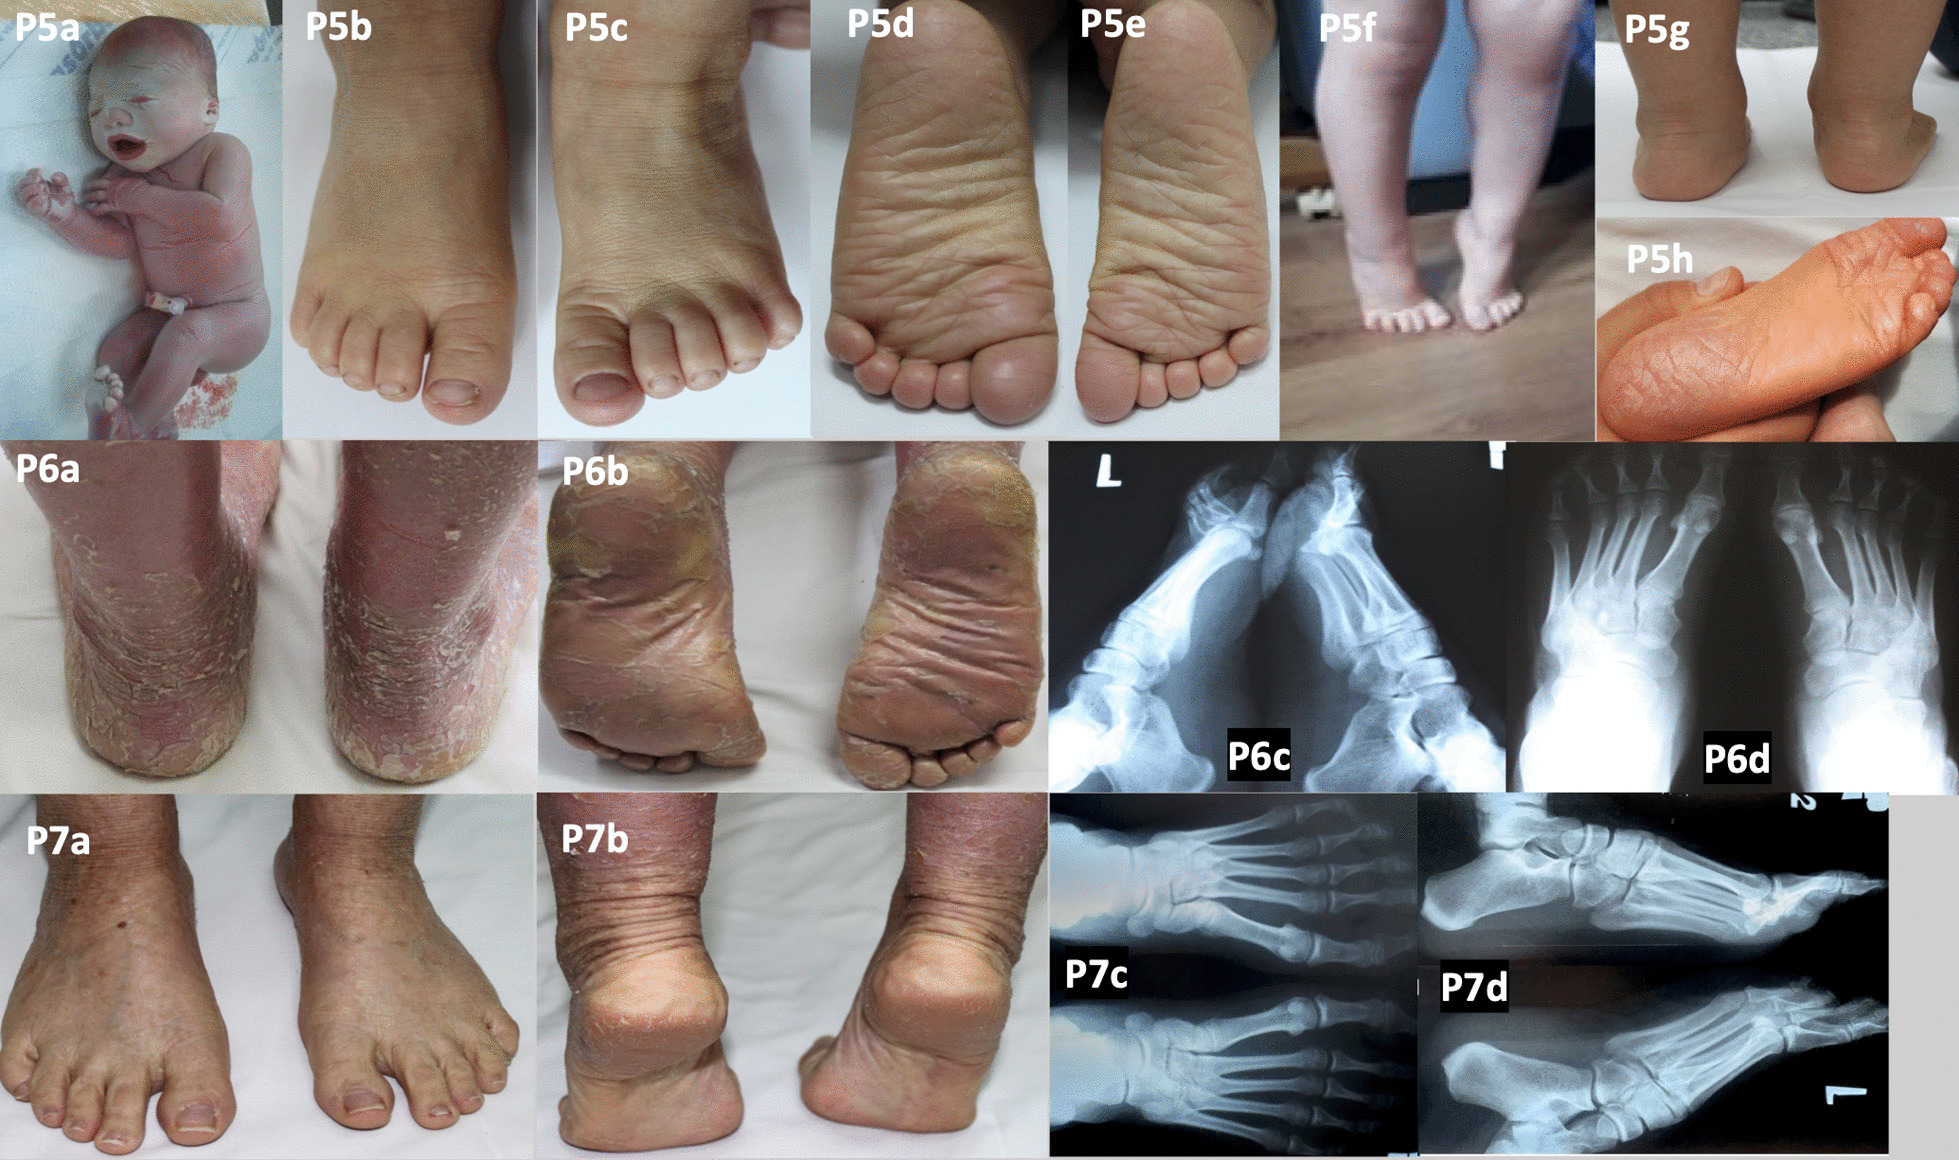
Patients 5–7 with autosomal recessive congenital ichthyosis. Collodion baby (P5a). Skin lesions on the dorsal aspect of the feet (P5b–c). Fissuring on the soles (P5d–e, h). Normal feet (P5f) and standing on tiptoes (P5g). Circular hyperkeratotic lesions on the ball of the foot, slight keratosis of the heels (P6a–b), clawed toes II–IV, and overlapping toes IV–V (P6c–d). Hyperkeratotic skin lesions on the soles (P7a–b). Normal X-rays of the feet (P7c–d)
